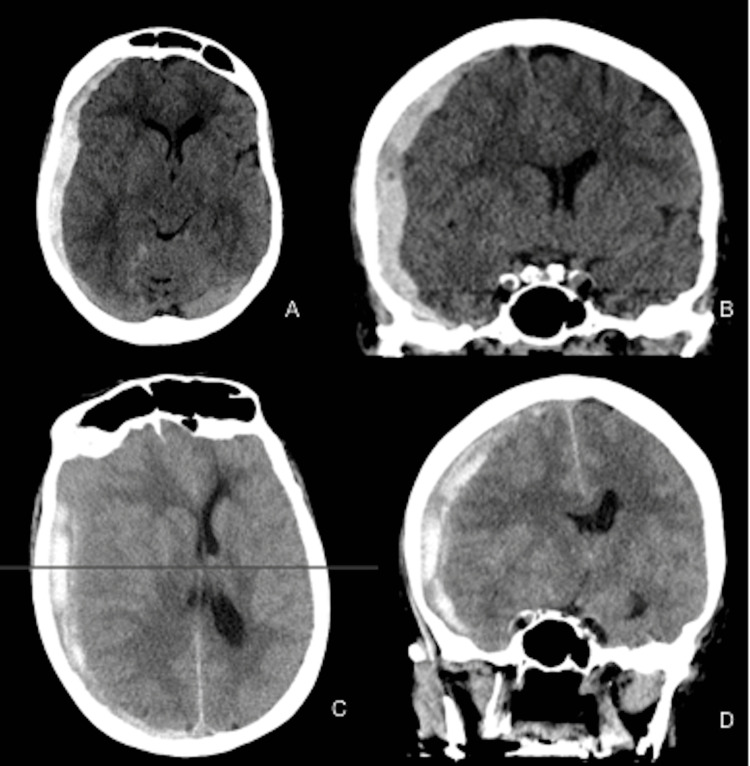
Computed tomography imaging of the head The top row shows axial (A) and coronal (B) views on the initial presentation. The bottom row shows axial (C) and coronal (D) views on re-presentation with acute neurological decline.
